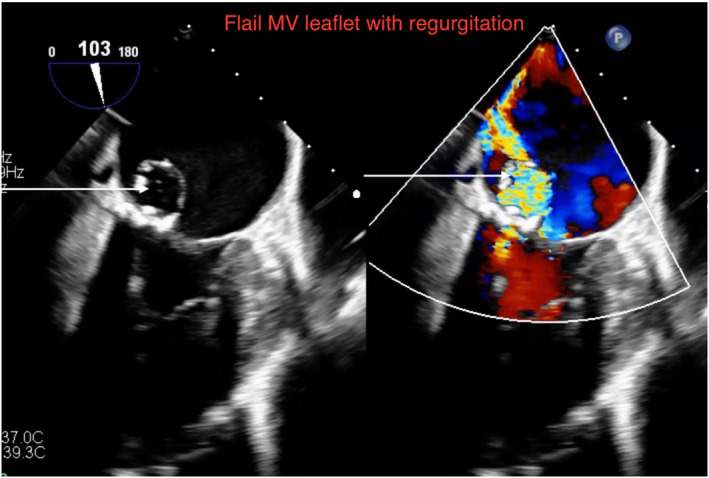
Echo showing flail MV leaflet with corresponding doppler showing regurgitation.
